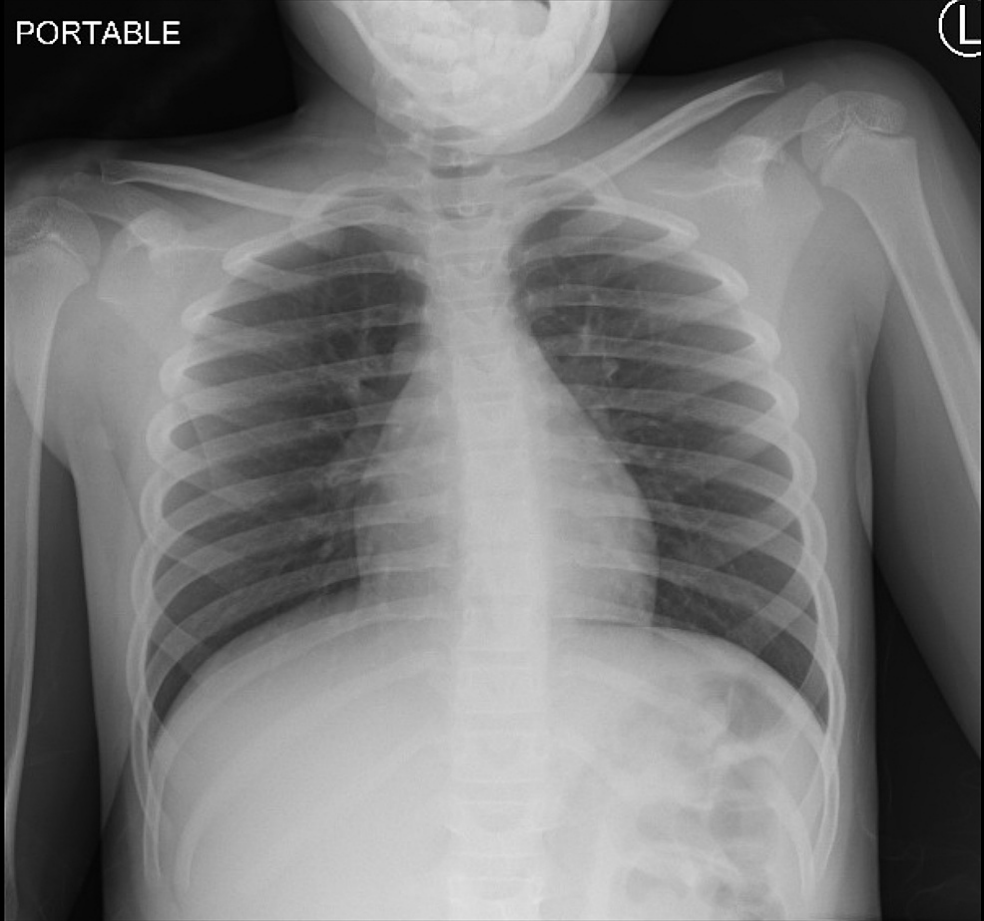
Normal CXR CXR: chest X-ray
